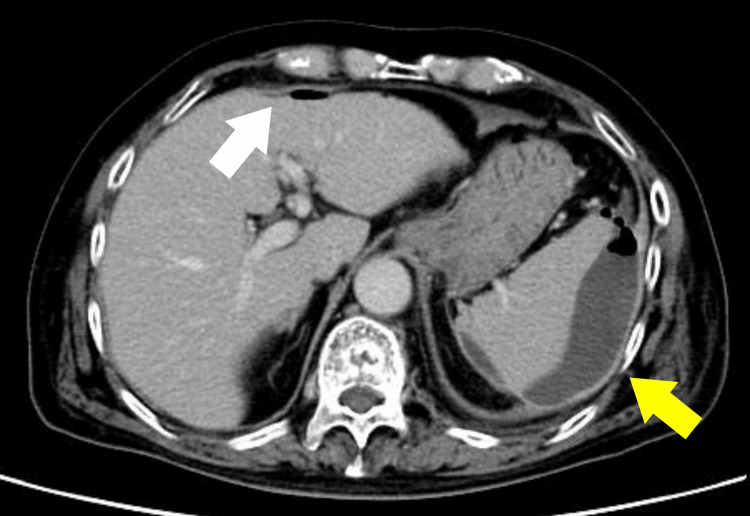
Contrast-enhanced CT at presentation showing a perisplenic abscess. An encapsulated fluid collection containing gas was seen extending from the perisplenic space to the left subphrenic space, consistent with a perisplenic abscess (yellow arrow). A small amount of free intraperitoneal air was also present along the liver surface (white arrow).
